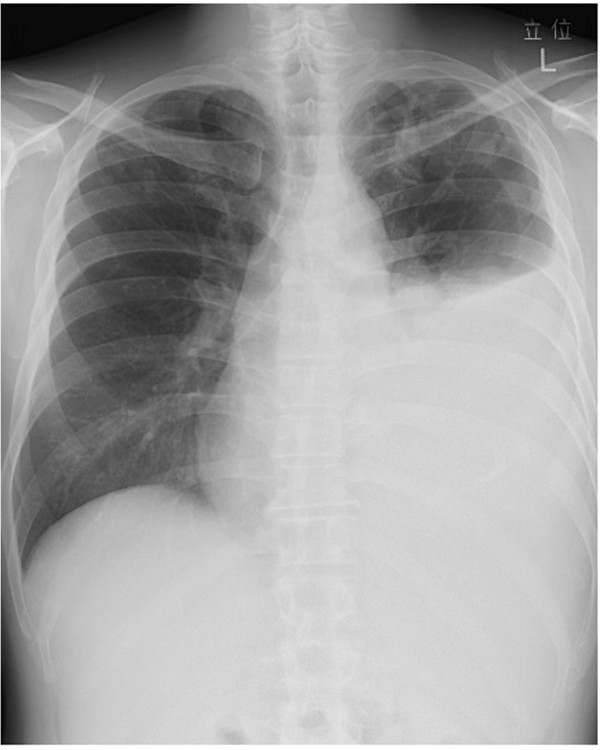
Chest X-ray in June 2007 revealed a left pleural effusion.
